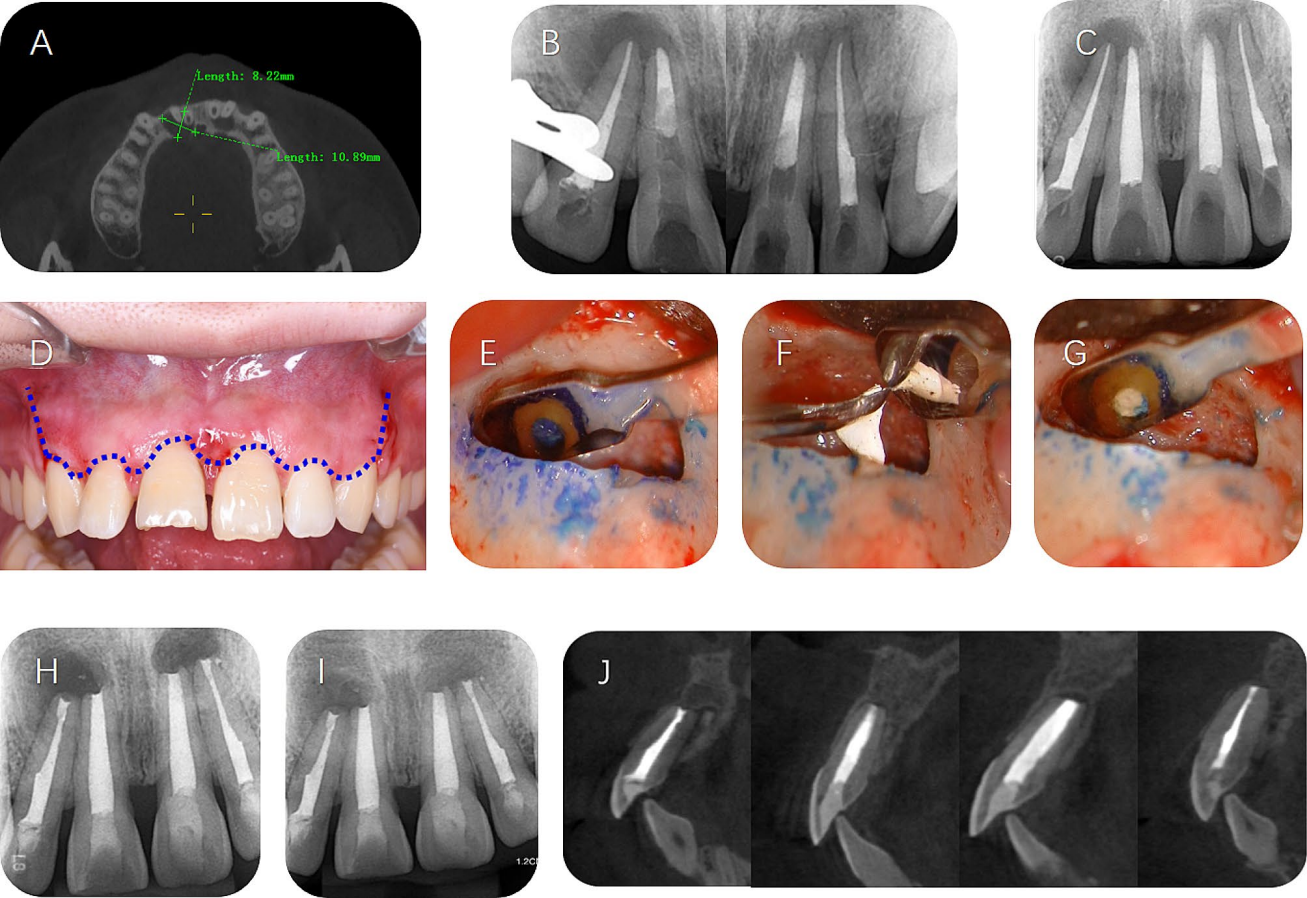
A representative case received the modified approach. (A) The CBCT scan revealed a lesion at the periapical region of the right central incisor and lateral incisor, and another independent lesion at the left central incisor and lateral incisor. (B) A periapical radiograph taken during root canal treatment indicated that the immature maxillary central incisors received orthograde obturated with iRoot-BP for 6 mm and filled with gutta-percha for the crown part, while two lateral incisors received obturation with gutta-percha and iRoot-SP by the warm gutta-percha obturation technique. (C) A periapical radiograph was taken at the 3-month follow-up. (D) A photograph taken before the surgery. The blue line represented the operative incision. (E), (F) and (G) The lateral incisors received retro-preparation and retro-filling with iRoot BP after apicoectomy. (H) A postoperative periapical radiograph was taken immediately. (I) Follow-up imaging showed partially healed of lesions 3 months after surgery. (J) A CBCT scan at the 12-month follow-up revealed healthy apical bone tissue around all roots
